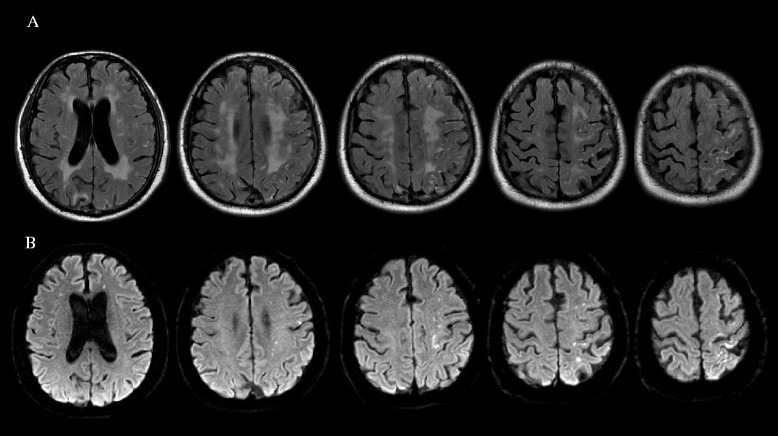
Immediate FLAIR MRI after Gd injection showed diffuse leptomeningeal enhancements along the cerebral cortex of the left hemisphere (A) accompanied by signal changes in DWI (B) in patient 1.
